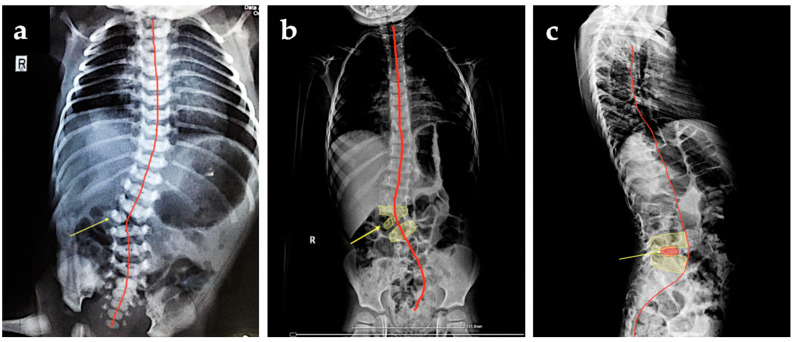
X-ray findings: (a) anteroposterior face of the child’s spine at 8 months after delivery indicating hemivertebra (yellow arrow); (b) anteroposterior X-ray face of the patient spine at 4-years-old depicting hemivertebra (yellow arrow) and scoliosis (red line), (c) lateral profile of the spine focused on the thoracolumbar section revealed the hemivertebra (yellow arrow), and curvature of the spine (red line).
